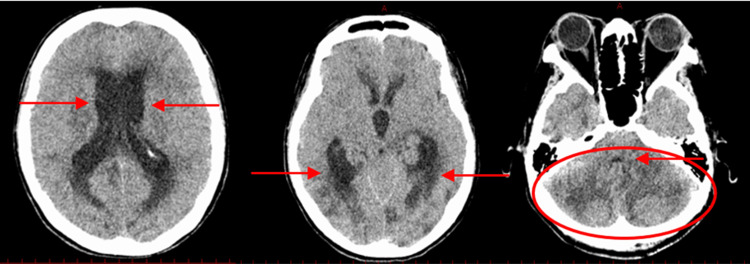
Non-contrast Brain Computed Tomography (CT) on Initial Examination Obstructive hydrocephalus with dilation of the third and lateral ventricles (red arrow).
